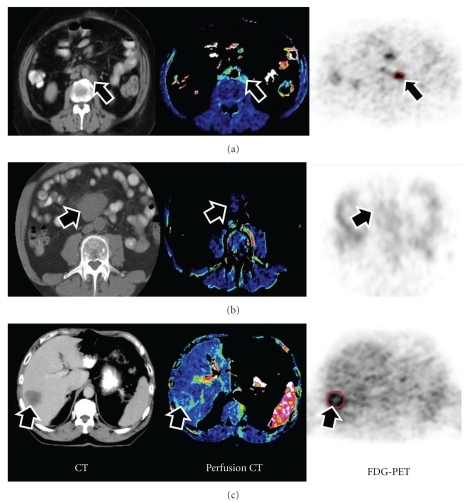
Conventional CT (left column), perfusion CT (middle column), and FDG-PET (right column) images of patients with (a) active lymphoma, (b) inactive lymphoma, and (c) colorectal liver metastases. Arrows indicate the tumour masses. Active lymphoma demonstrated high blood flow and high FDG uptake, inactive lymphoma, low blood flow and low FDG uptake, whilst the colorectal liver metastasis demonstrates low blood flow and high glucose metabolism.
